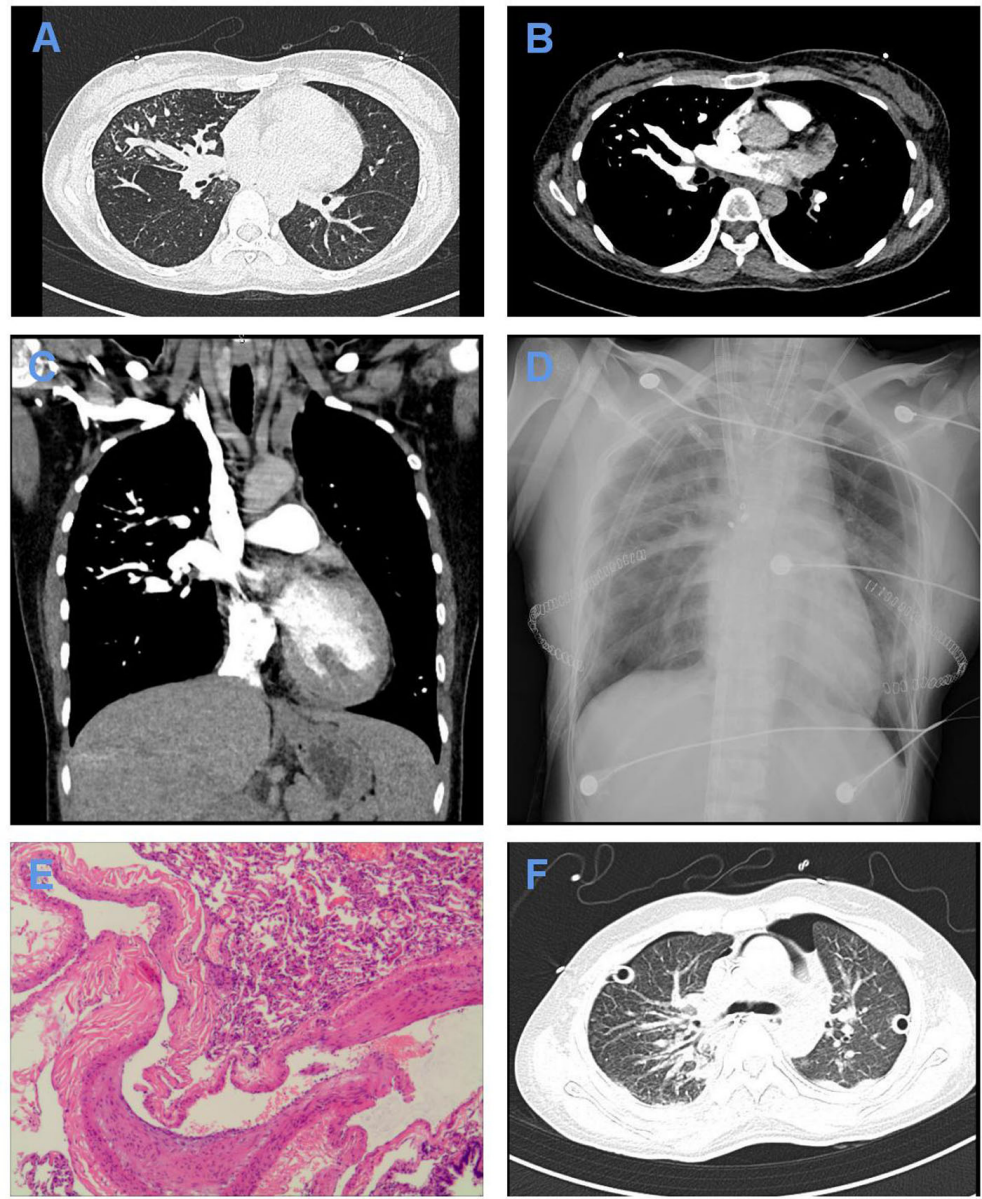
Imaging and pathological data of the patient. (A–C) Lung window (A), mediastinal window (B), and 3D window (C) of CTPA after admission showing simultaneous filling of the pulmonary artery and pulmonary vein in the right lung and the lower lobe of the left lung fill. (D) X-ray of the chest on the first postoperative day showing exudative changes in both lungs. (E) Pathological examination showing dilated blood vessels, different thicknesses of the blood vessel wall, partial anastomosis of the dynamic venous blood vessel wall, thickening of the intima of some blood vessels, and congestion and bleeding in the lung, conforming to the standard of PAVF with hemorrhage and secondary pulmonary hypertension. Scale: ×40. (F) Chest CT showing a small amount of pleural effusion on both sides, slight enlargement of the hilum of both lungs after double-lung transplantation, interstitial changes in both lungs with exudation, local pericardium thickening, and locally thickened pleura on both sides.
